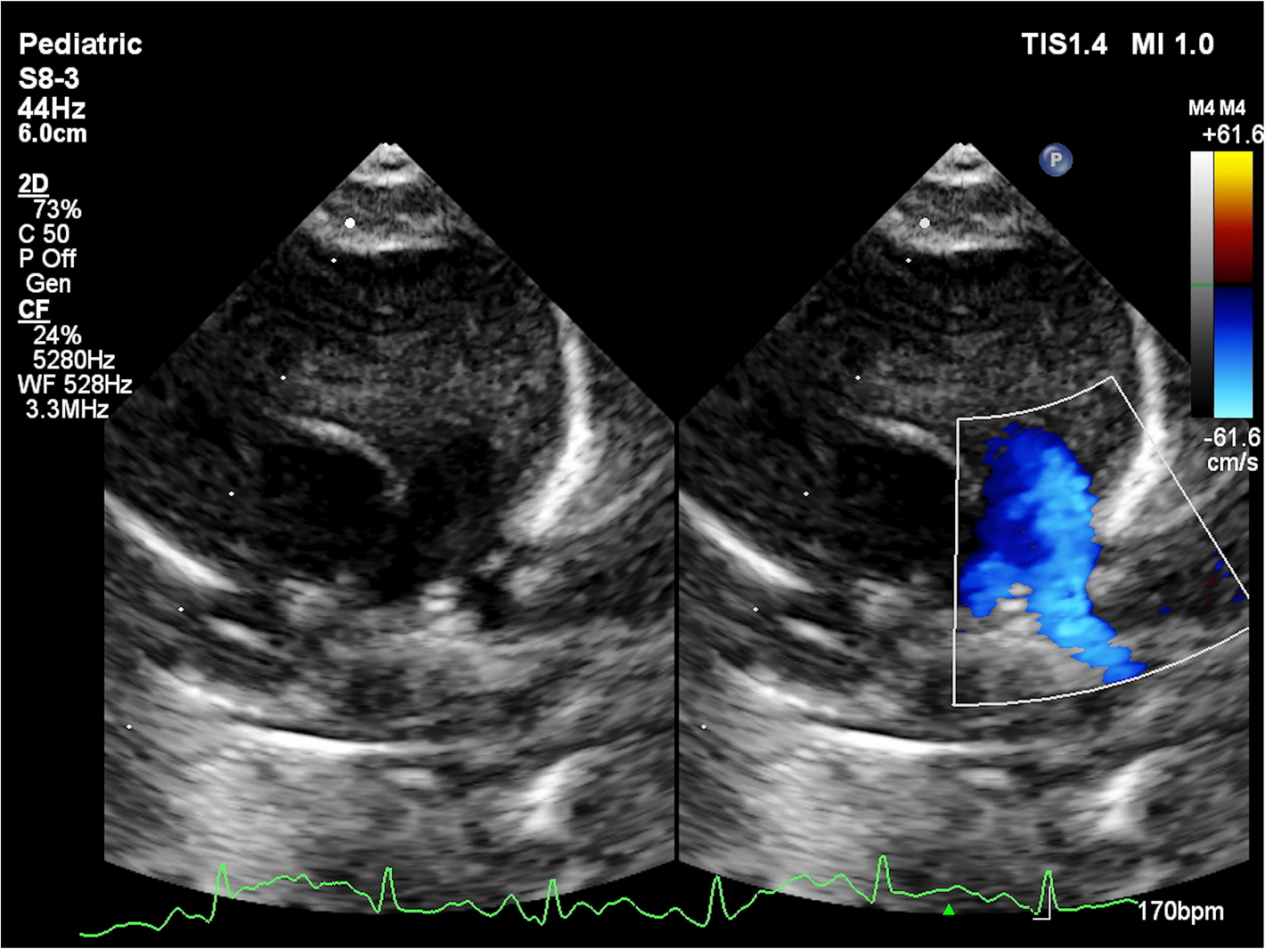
Repeat echocardiogram after 6 weeks of LMWH. Colour Doppler in parasternal short axis view showing partial resolution of thrombus in LPA (arrow)
